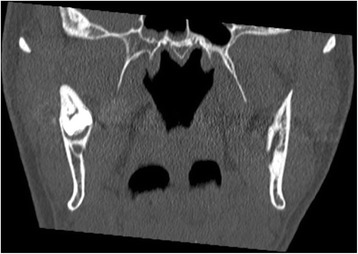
Coronal cone beam scan showing the impacted tooth with radiolucent cystic lesion superior the inferior alveolar nerve
